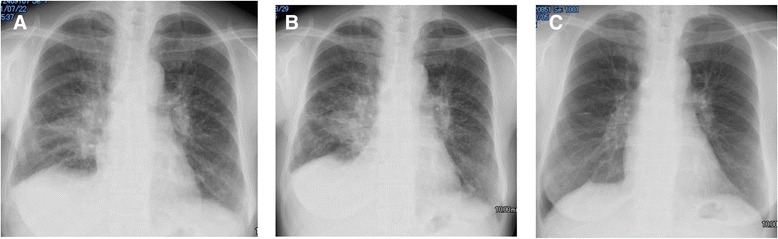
Chest radiography. a Before the first admission. b Before the initiation of corticosteroid therapy. c Five weeks after the initiation of corticosteroid therapy
